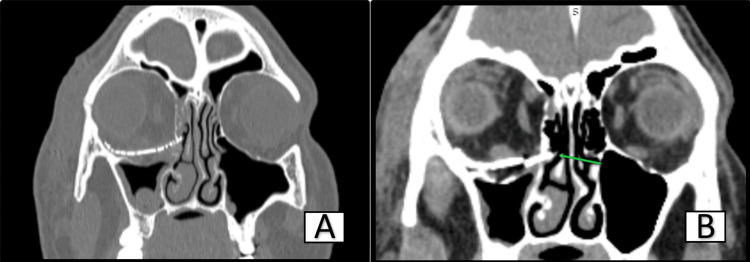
HRCT coronal section showing overextension of orbital mesh medially (green arrow) HRCT: High-resolution computed tomography
